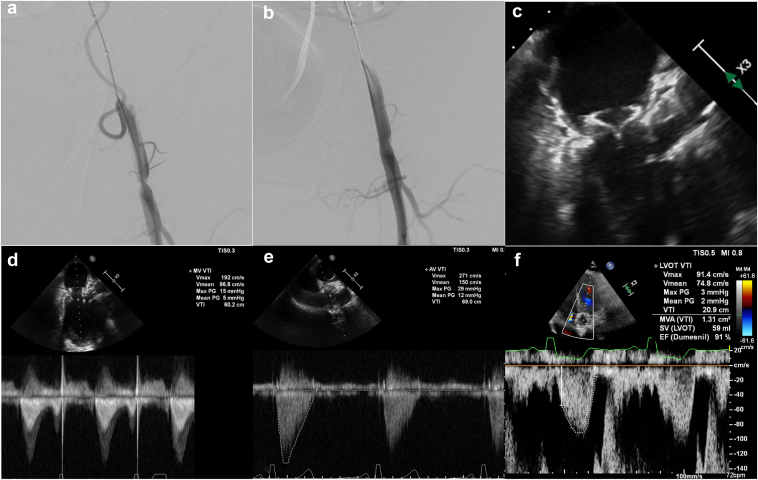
Vascular access management, procedural and follow-up results. (a) Angiography of the main transcatheter aortic valve replacement access via the cross-over balloon showing significant residual stenosis after vascular closure devices were closed. (b) Result after balloon angioplasty using the same premounted over-the-wire balloon from the contralateral femoral artery. (c–e) Postprocedural TEEevaluation showing: c – good valves position and function with no mechanical obstruction of the LVOT; d – mean mitral valve gradient of 5 mmHg; e – mean aortic valve gradient of 12 mmHg. (f) One-month follow-up transthoracic echocardiogram still showing no signs of LVOT obstruction. Abbreviations: LVOT, left ventricule outflow tract; TEE, transesophageal echocardiography.
